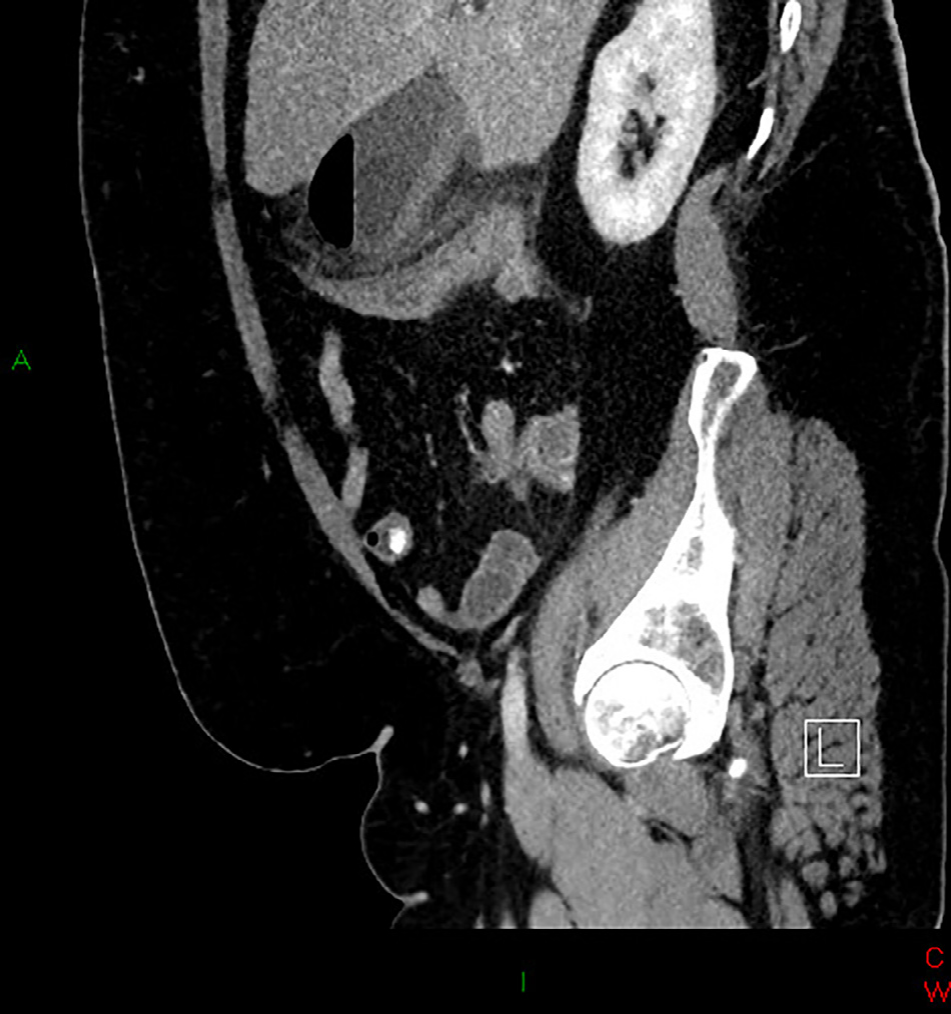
A contrast-enhanced CT scan sagittal plane showing acute emphysematous cholecystitis. Air-fluid level in the lumen of gallbladder with wall thickening, increased enhancement in the gallbladder wall, inflammatory stranding of surrounding fat planes.
